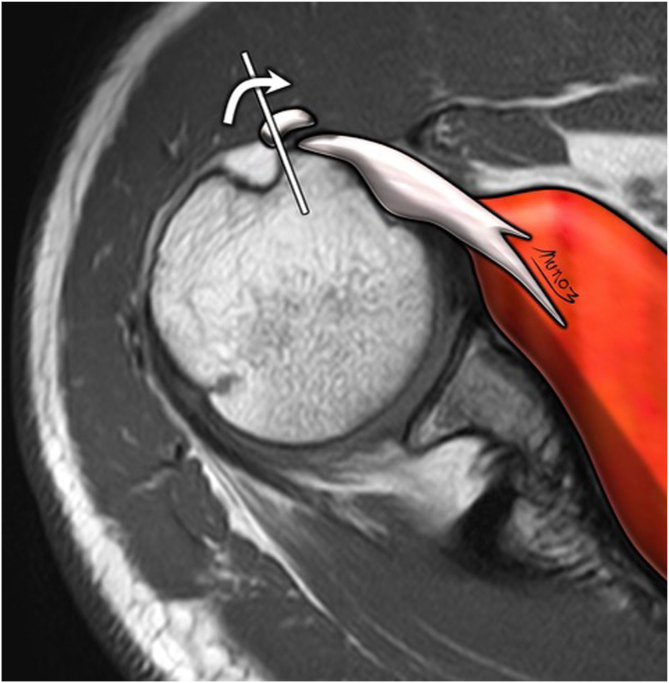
LHBT subluxation sign is shown in an axial view of a T1-weighted right shoulder MRI. The white line marks the limit of the bicipital groove. The white curved arrow shows the direction of a subluxed or displaced LHBT from the bicipital groove. (LHBT, long head biceps tendon; MRI, magnetic resonance imaging.)
